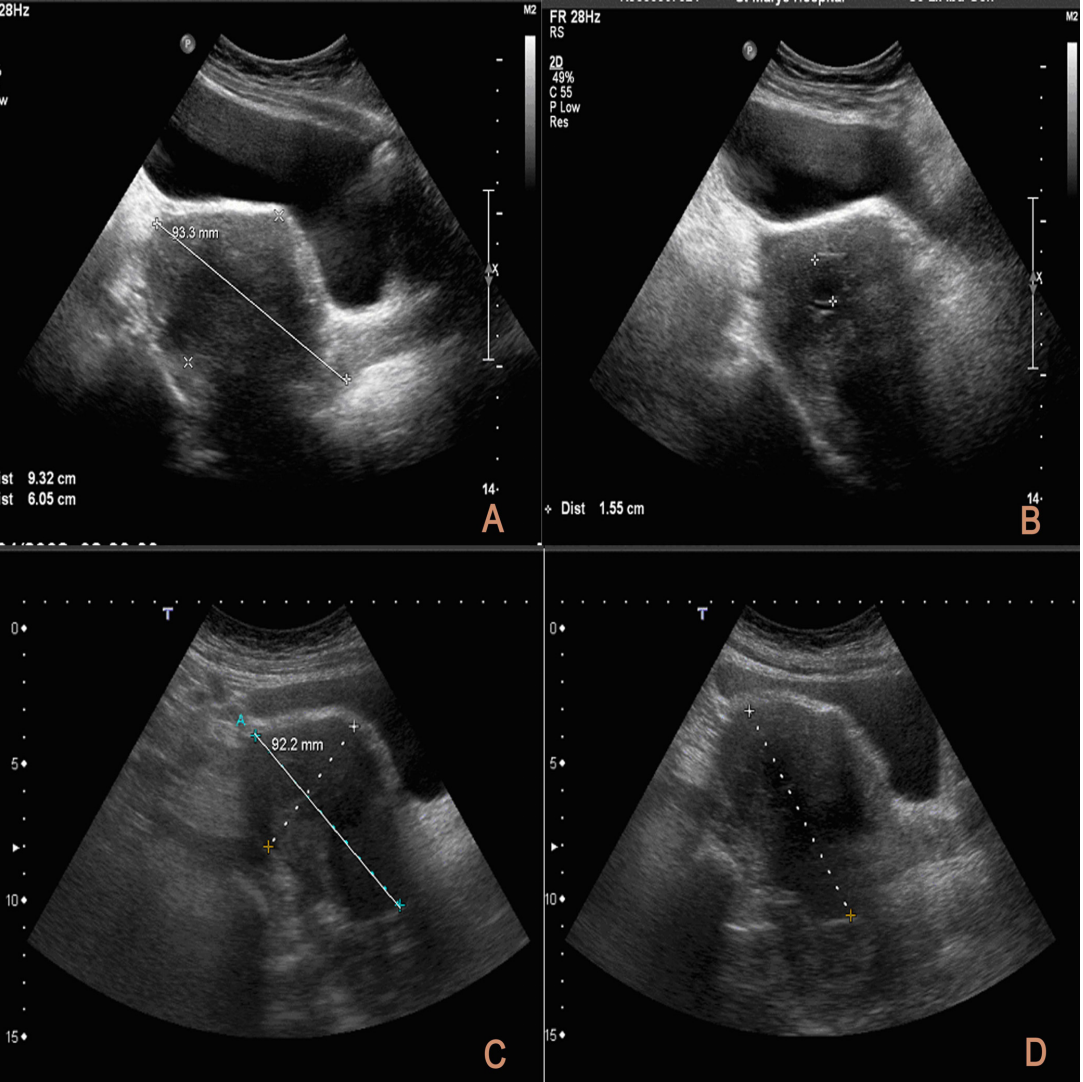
Ultrasound (U/S) of the pelvis. (A) 1 year after the diagnosis showing a reduced size (93.3 mm) hypo-echoic ovarian mass and resolution of the ascites. (B) 2 years after the diagnosis showing the unchanged ovarian mass and an irregular necrotic area in the centre (also present on previous scans). (C/D) 3 years after the diagnosis showing no progression and rather an improvement of the disease.
